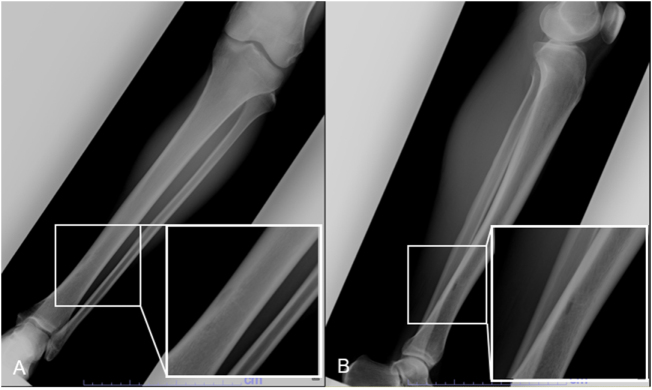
Anteroposterior (A) and mediolateral (B) radiograph showing discontinuous osteolytic lesions in the tibia.
